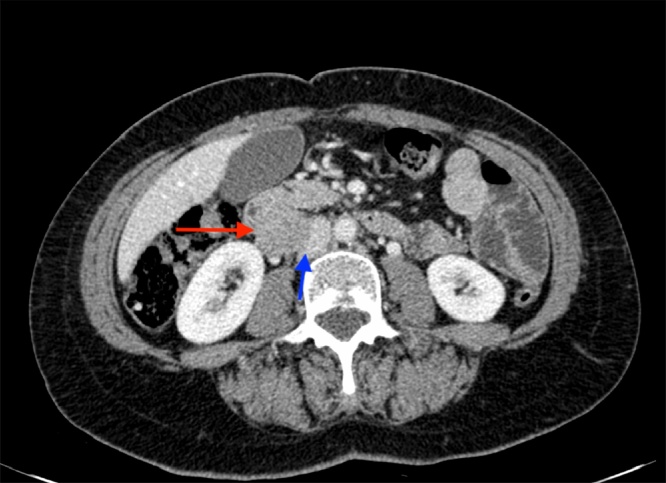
A contrast enhanced abdominal CT scan image. Red arrow pointing to the leiomyosarcoma and blue arrow pointing to the compressed IVC (For interpretation of the references to colour in this figure legend, the reader is referred to the web version of this article).
